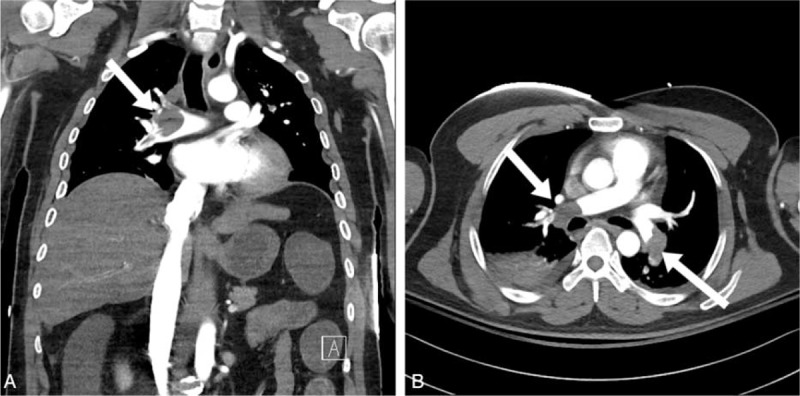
Chest computed tomography scan showing extensive bilateral pulmonary embolism in both pulmonary arteries. (A) Coronal image, (B) axial image.
